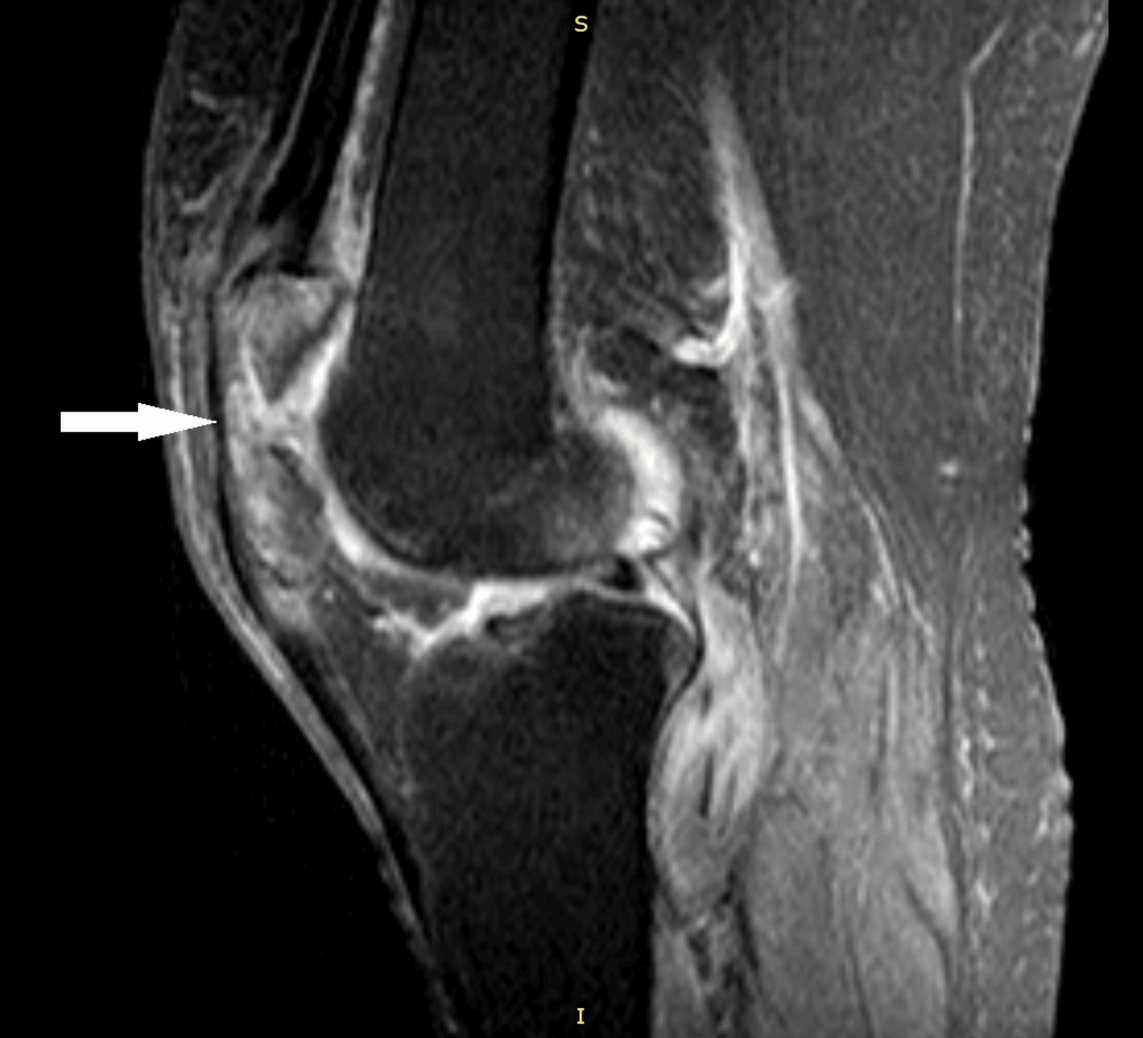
Sagittal view T2-weighted proton density inversion recovery MRI showing increased signal surrounding patellar fragments demonstrating inflammation.
